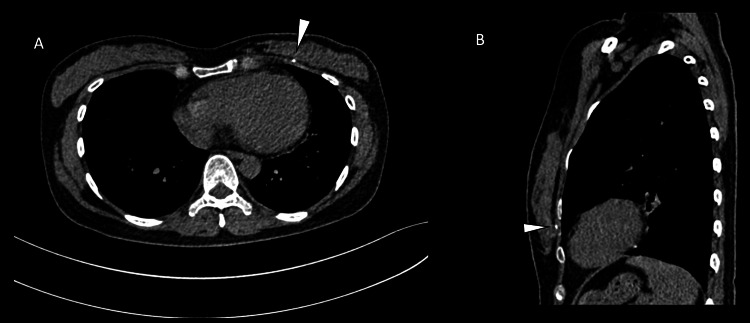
CT-guided fluoroscopy in axial (A), sagittal (B) shows a dense foreign object (white arrowheads) lying posterior to the left pectoral muscle. Due to its compromising position, the decision to insert a hook wire was not taken.
